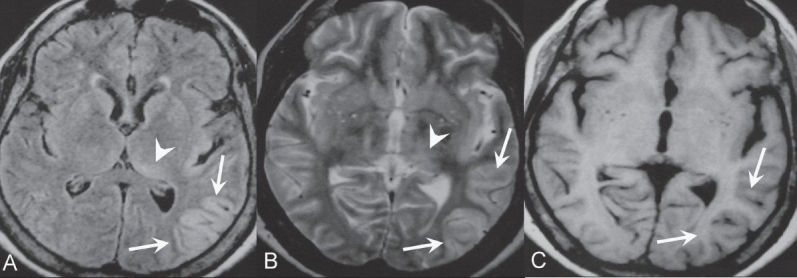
Axial FLAIR (A) and T2W (B) images demonstrate gyriform hyperintensity in the left parietal and insular cortex (arrow), which appears hypointense (arrows) on the axial T1W image (C). Note the ill-defined hyperintensity in the left thalamus (arrowhead in A and B)
